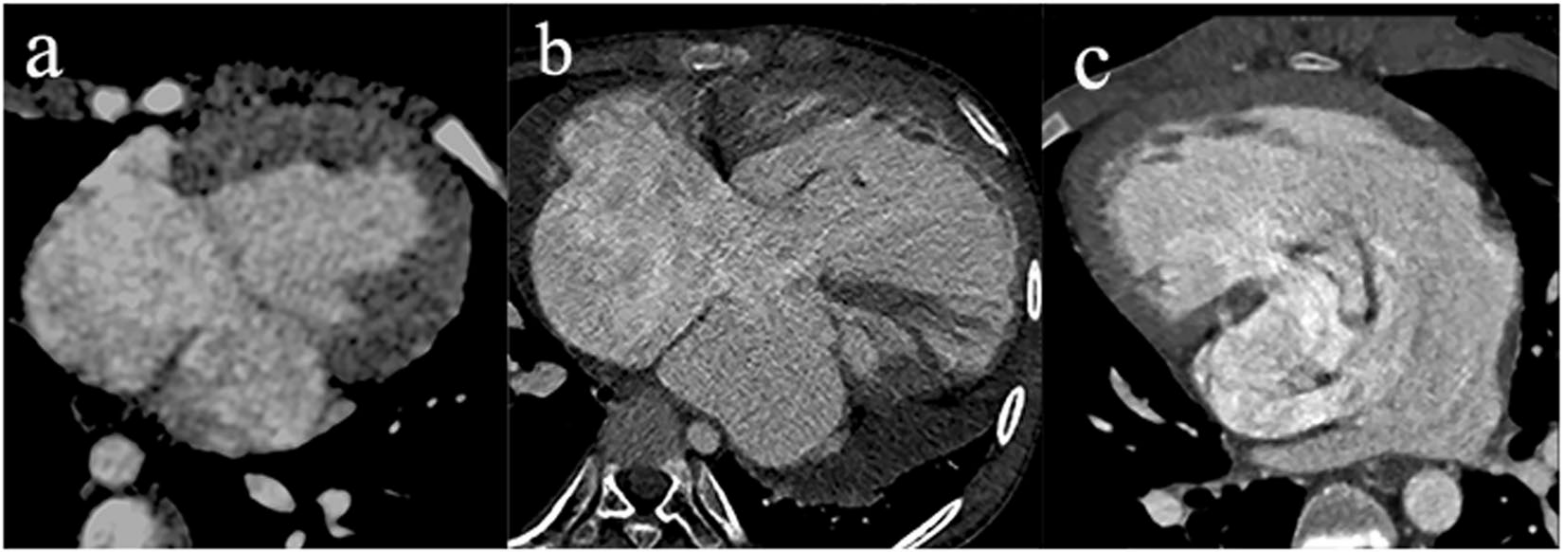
Anatomic types of SV demonstrated by DSCT. Single left ventricle. (a) The ventricle is characterized by relatively smooth walls, fine trabeculations and lack of septal chordal attachments of the atrioventricular valve. Single right ventricle. (b) The ventricle has more coarse trabeculations always accompanied with chordal attachments to the septal surface. Undifferentiated ventricle. (c) The ventricle has the features which are hard to divide into the two types mentioned above. DSCT indicates dual-source computed tomography; SV, single ventricle.
